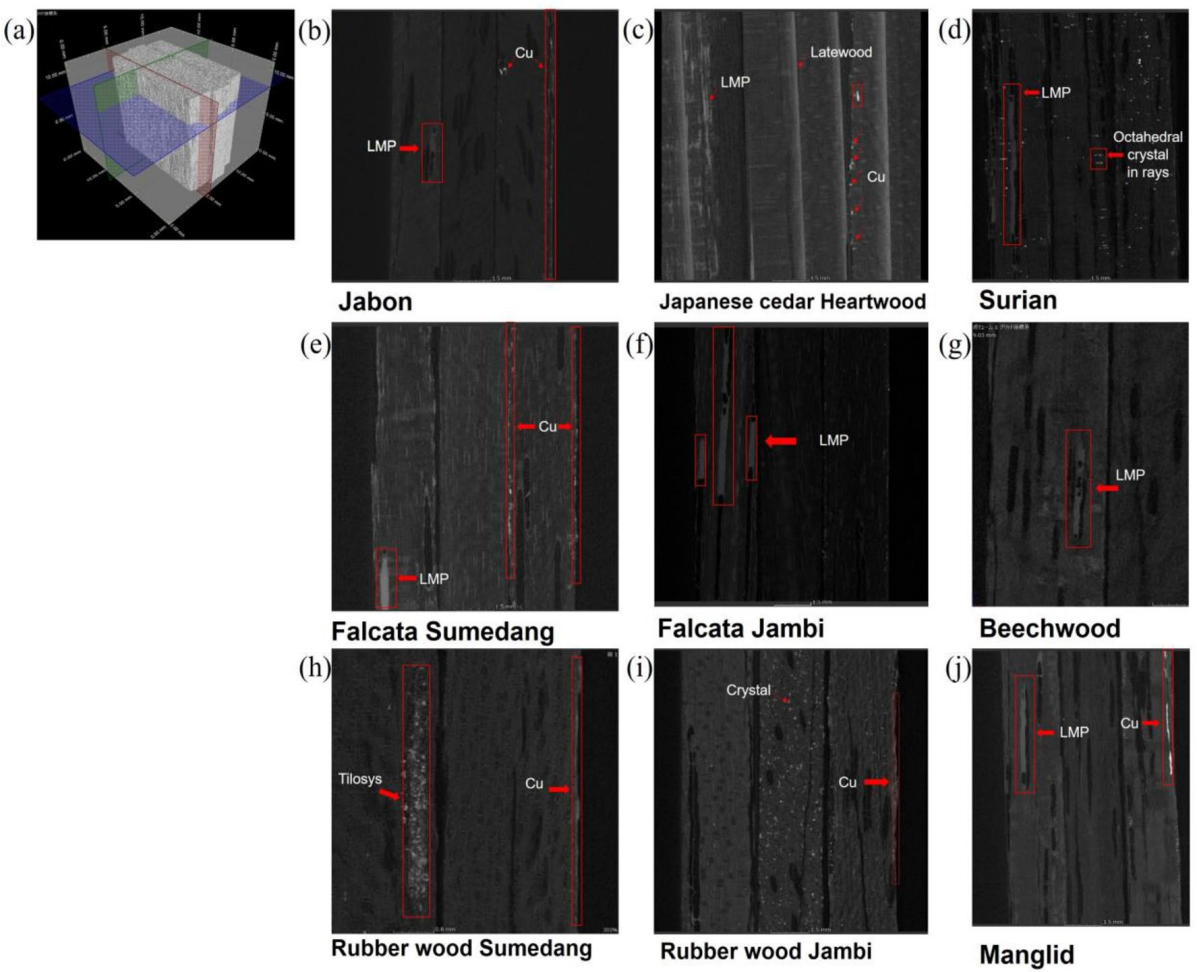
A 3D X-ray observation of the distribution of LMP and Cu. (a) A 3D image stack of the treated-veneer by LMP and copper naphthenate, and the untreated veneer; softwood and hardwood 2D representative images of a radial section were obtained from the green cut section of the 3D image, (b) Jabon wood from Sumedang city, (c) Japanese cedar heartwood veneer, (d) Surian wood, (e) Falcata wood from Sumedang city, (f) Falcata wood from Jambi city, (g) Beechwood, (h) Rubber wood from Sumedang city, (i) Rubber wood from Jambi city, and (j) Manglid wood.
